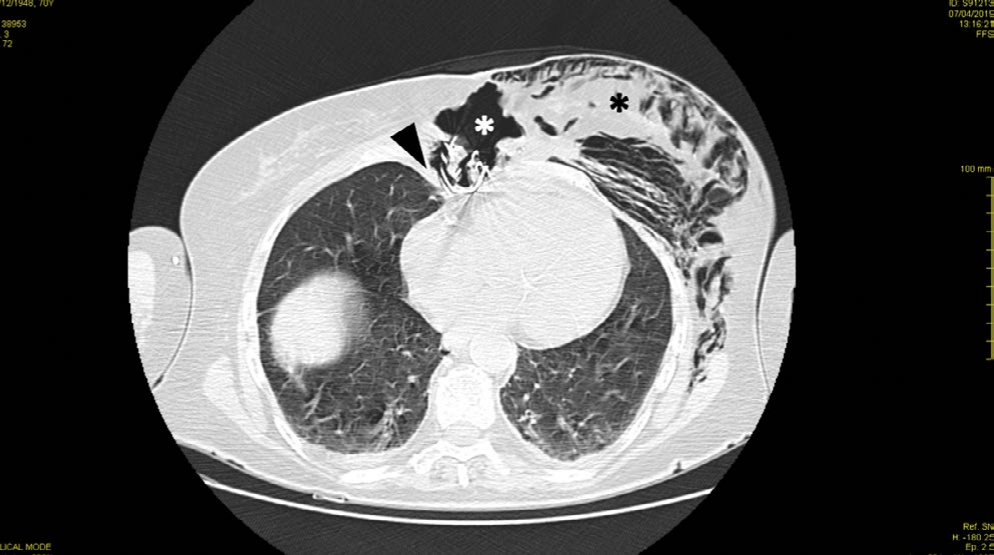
Computed tomography scan revealed pneumomediastinum with air passing through the sternum to the left hemithorax (*). The sternal bone was fractured, and the right steel wire had tight relation with the right middle lobe (arrow)
